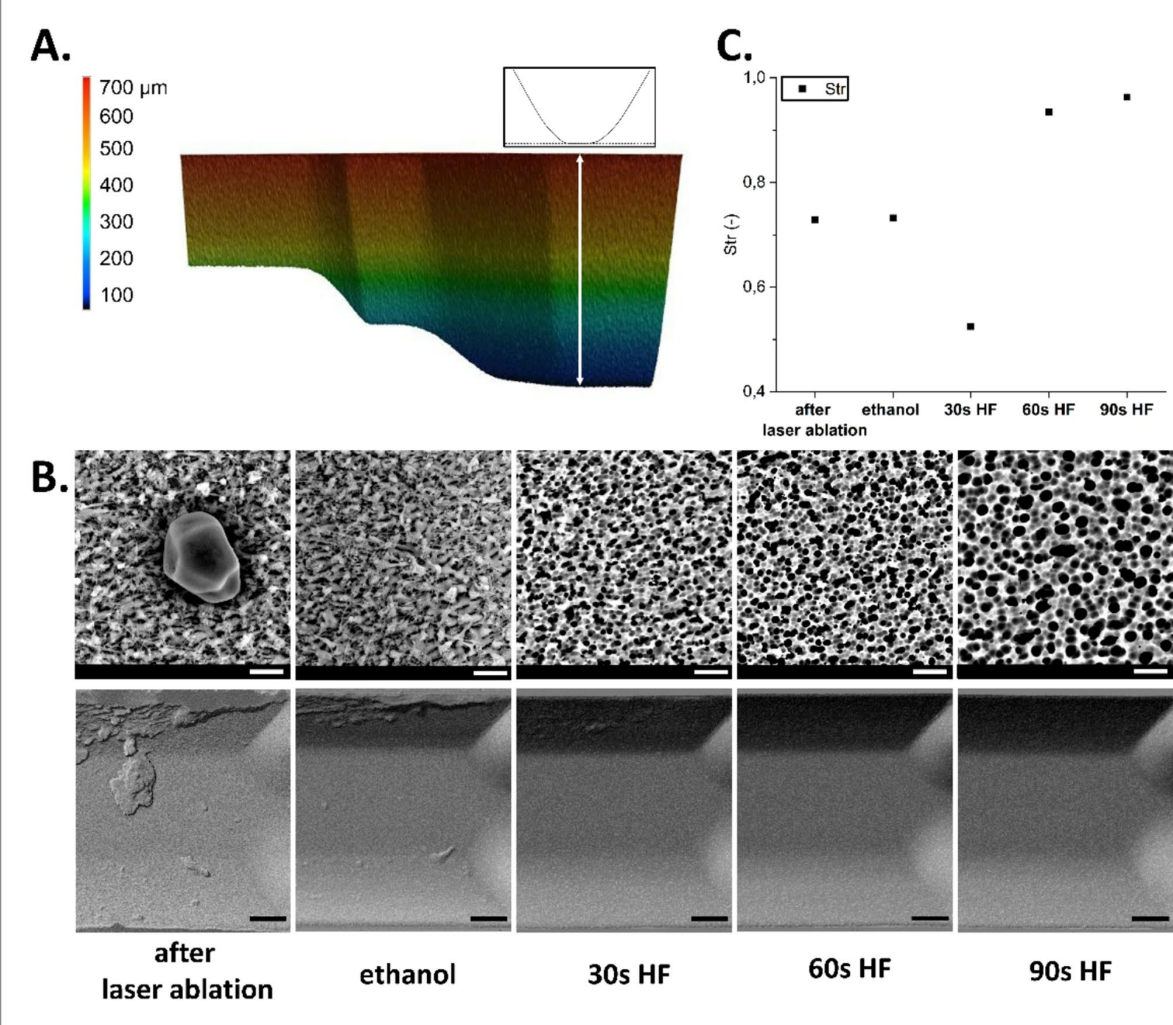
Post treatment after laser ablation including chemical cleaning. (A) Cross sectioned view of the evaluated complex system design with corresponding cross sectional line profile, that was used for roughness measurements. The roughness after 14 layers of 50 μm height ablation equals Sq = 1.73 μm. (B) SEM images (upper row) and DIC images (lower row) of laser structured parameter slots surfaces after subsequent chemical treatment steps. The first image shows the surface of the glass right after the laser ablation. For the second image, glass was cleaned in an ultrasonic bath filled with ethanol for 15 min to wash away the debris. For the following images, the glass was dipped in a glass-etching solution for 30 s, 60 s and 90 s resulting in typical surface pits. The scale bar is 5 μm for SEM and 200 μm for DIC images. (C) The roughness values (Str) on glass surfaces measured after the individual glass treatment steps. For a surface with a dominant lay, the Str parameter tends towards 0, whereas a spatially isotropic texture results in a Str close to 1. The progressing chemical treatments can selectively dissolve or wash away certain components of the glass, leading to specific surface features that can either increase or decrease surface roughness, depending on the treatment used.
